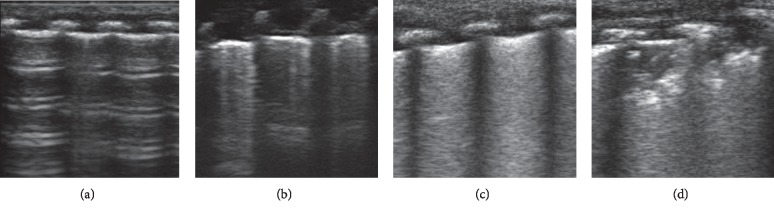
Four ultrasound aeration patterns. (a) Normal aeration (N): the presence of lung sliding with only A-lines or fewer than three isolated B-lines; (b) moderate aeration loss: interstitial syndrome, localized pulmonary edema, defined by coalescent B-lines in less than 50% of the intercostals space, multiple well-defined B-lines, or subpleural consolidations; (c) severe aeration loss: alveolar edema, defined by diffused coalescent B-lines occupying the whole intercostals spaces; (d) complete aeration loss (C): the presence of a tissue pattern with or without air bronchogram.
